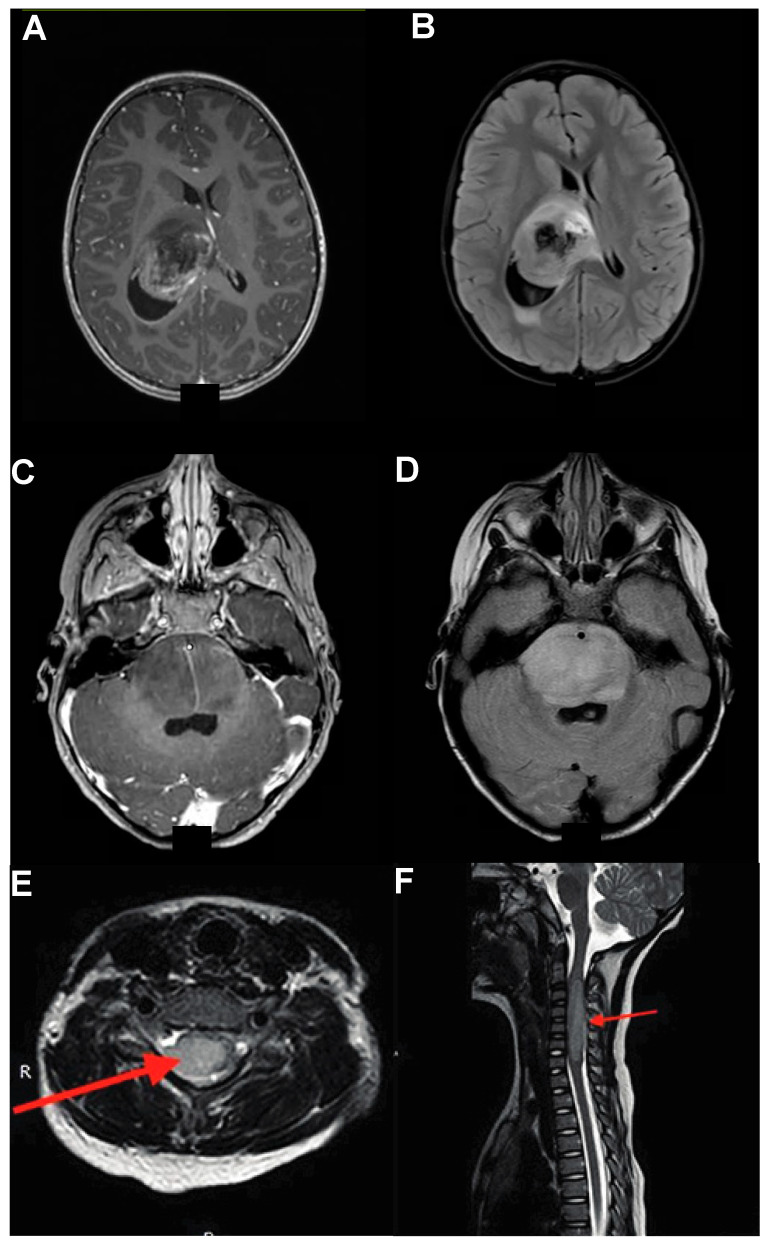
DMGs Imaging Characteristics. (A,B) Thalamic gliomas show involvement of various thalamic nuclei (A) and a significant FLAIR signal (B) causing locoregional mass effect, ventricular entrapment, and internal capsule involvement. (C,D) DIPG and midbrain DMGs are characterized by wispy heterogeneous contrast enhancement (C) and enlargement of the pons (D). (E,F) Spinal gliomas, like their cranial counterparts, are characterized by heterogenous contrast enhancement (E), with significant cranio-caudal spread (F). Adapted from [31].
